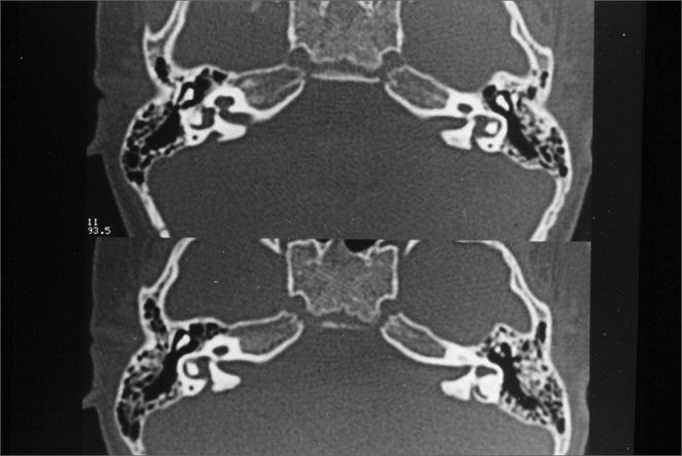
Bilaterally enlarged vestibular aqueduct - temporal bone CT scan - axial view.
